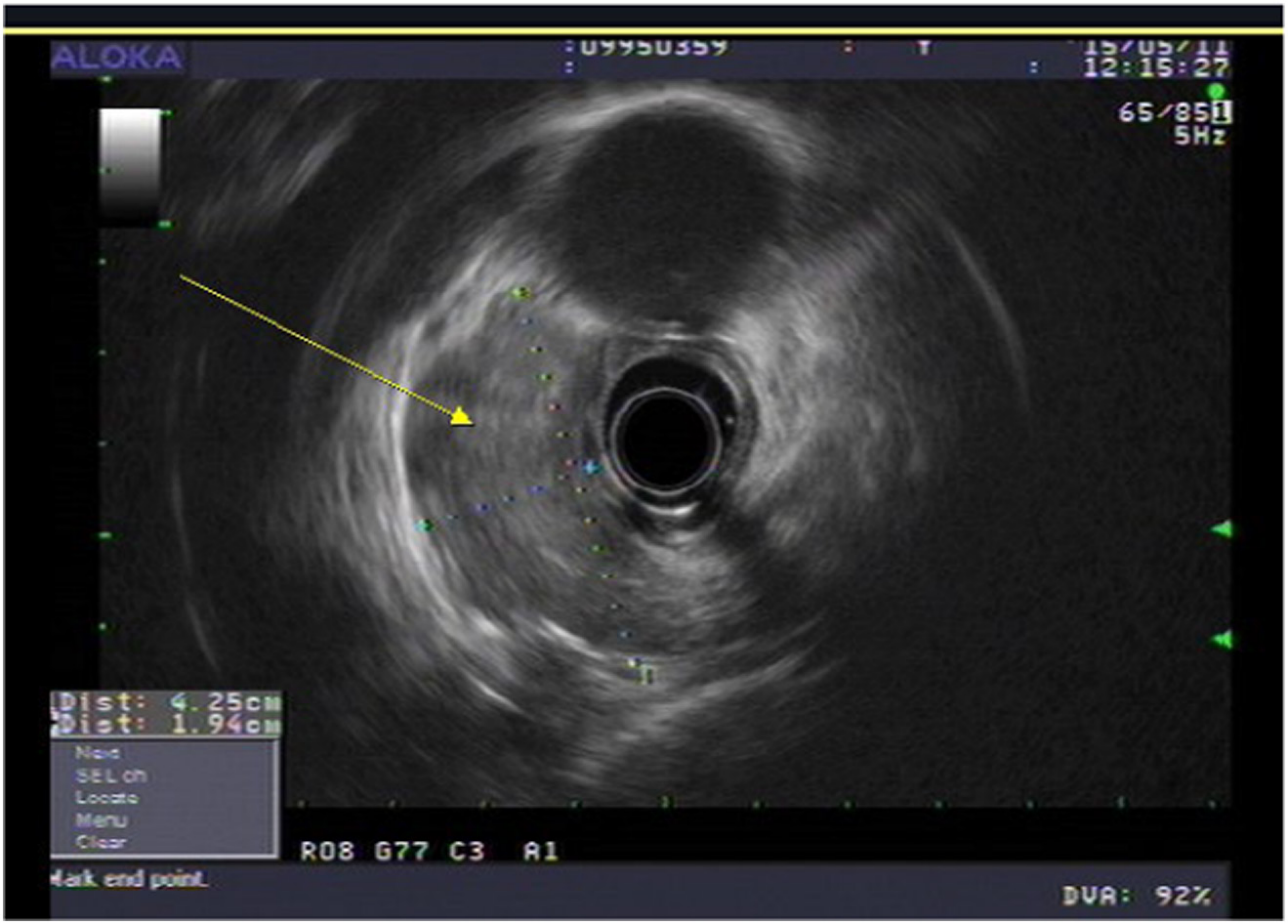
Endoscopic ultrasound (EUS) endoscopic ultrasound presented a 43 × 23 mm‐sized cancer lesion ranging from UI 33 cm to 29 cm. On EUS, the adventitia layer could not be distinguished, and esophageal invasion was strongly suspected as focal discontinuity of the SM layer and thickening of the PM layer were observed. (arrow).
